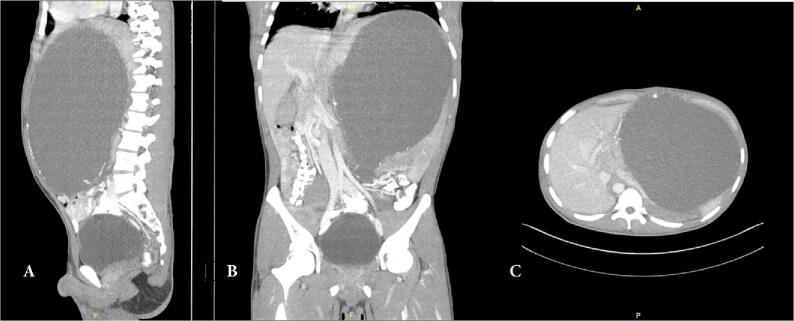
(A) The longitudinal plane (B) the coronal plane (C) and the transverse plane of a contrast abdominal CT scan showed a splenic cystic formation, rounded and with a well-defined border.
